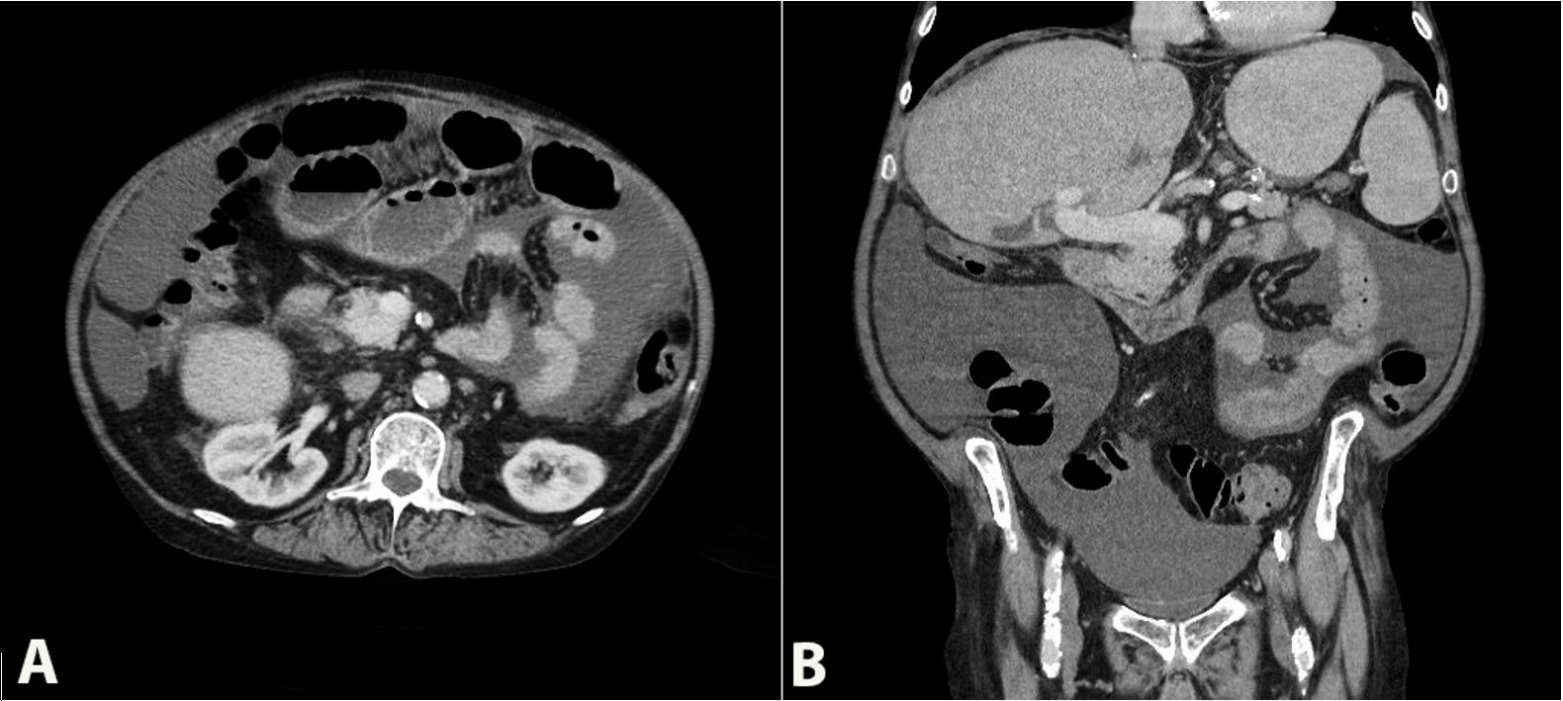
Abdominal CT Scan revealing diffuse dilatation of small bowel’s loops in the (A) axial plane and (B) coronal plane.
